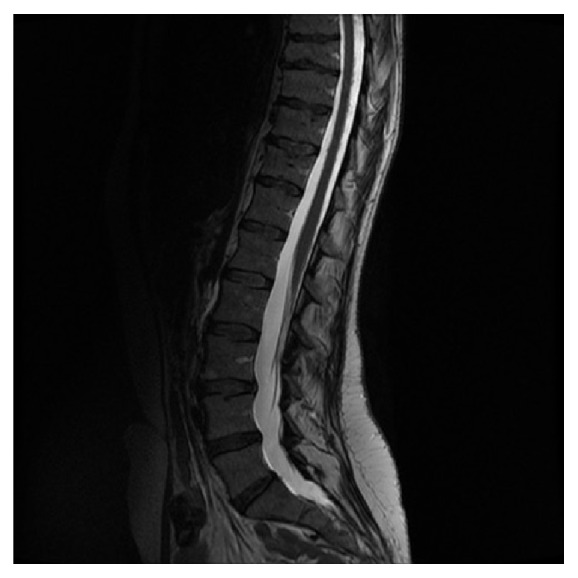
Spine MRI, lumbosacral region.
